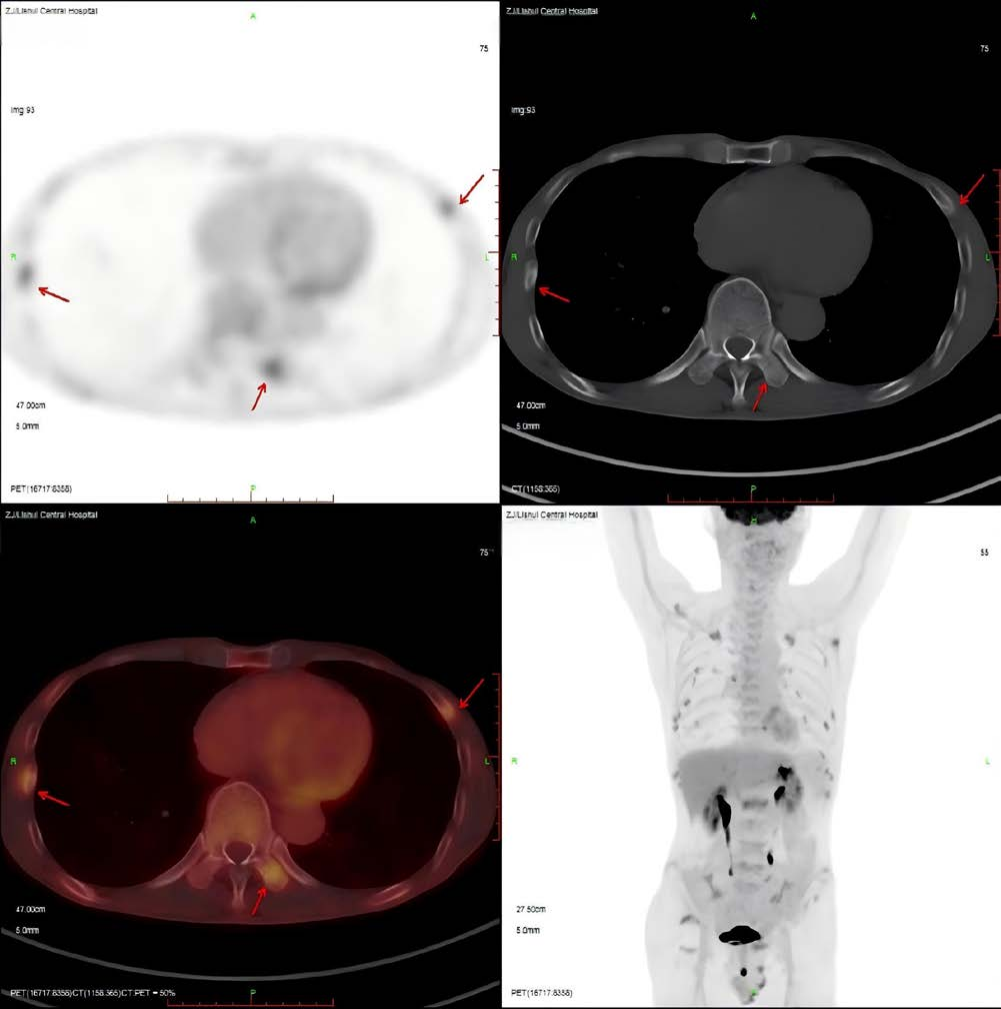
Positron emission tomography-computed tomography.
